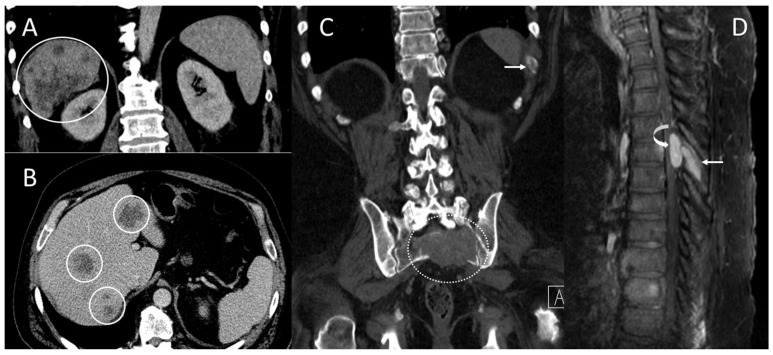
Computed tomography (CT) scans and magnetic resonance imaging (MRI) scans at initial diagnosis showed metastatic lesions in the liver, left 11th rib, 6th thoracic spine (T6), and sacrum. (A) Coronal and (B) axial contrast-enhanced CT scans demonstrate multifocal heterogeneous irregular hepatic tumors of varying sizes throughout the liver (solid circles) primarily in the right hepatic lobe. (C) Coronal CT scans in bone window demonstrate a lytic bone metastasis involving the left eleventh rib (arrow) and a large, destructive upper sacral metastasis (dashed circle). (D) Sagittal contrast-enhanced MRI scans demonstrate an enhancing mid-thoracic spine epidural metastasis (curved arrow) with an adjacent bone metastasis involving the T6 spinous process (arrow).
